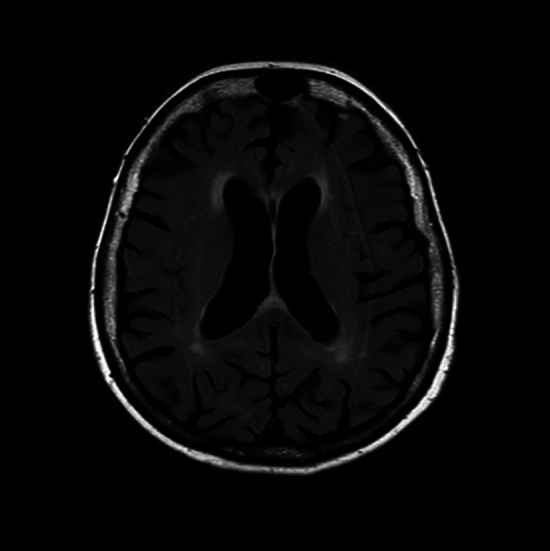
MRI demonstrating white matter hyperintensities (WMHs) with no features of stroke or intracranial bleeding
